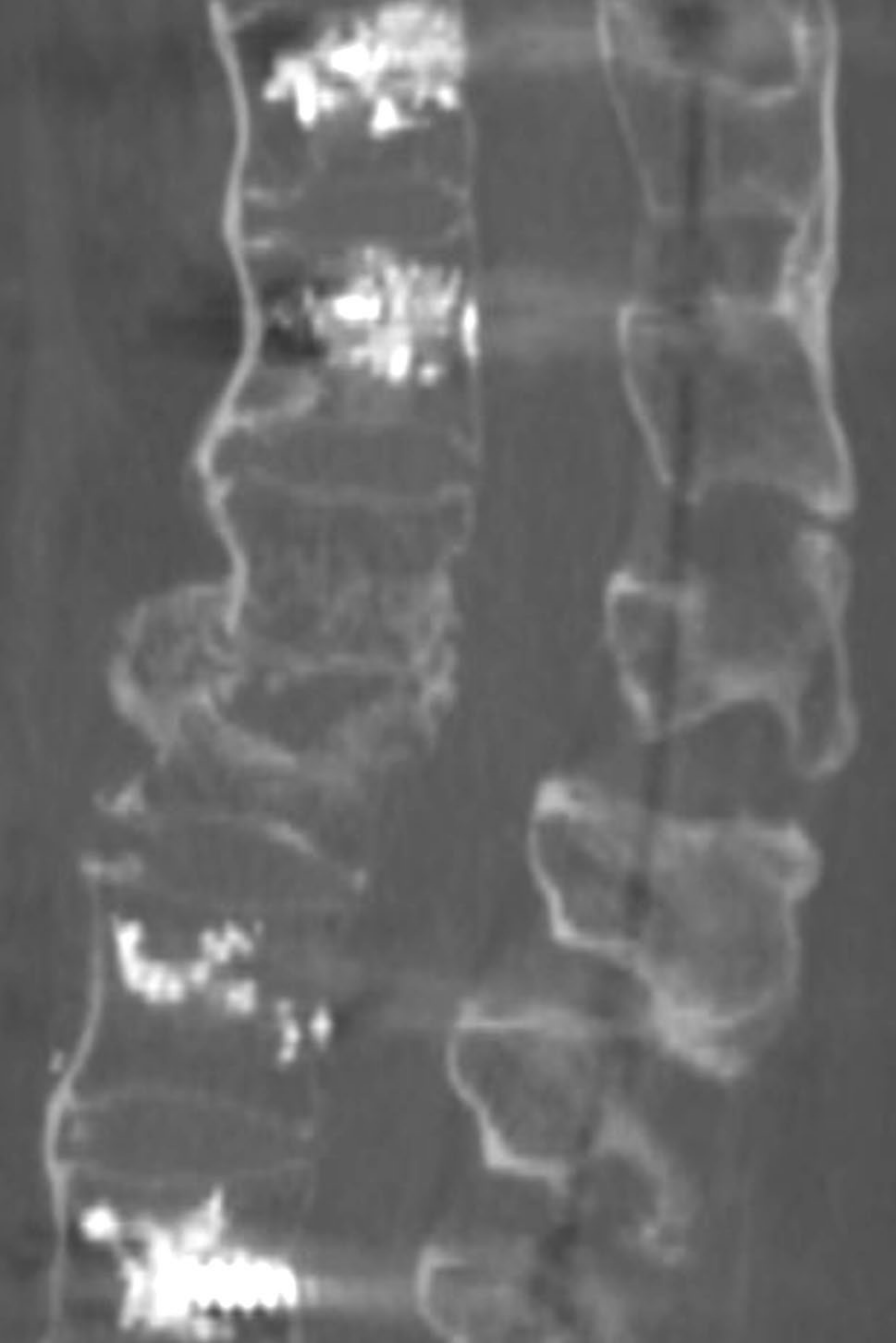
CT scan after 6 months showing osseous healing of the fracture
